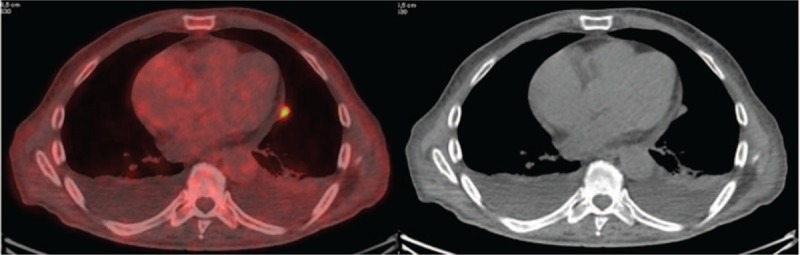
^18^F-FDG-PET/CT scan: small uptake in the left paracardiac area at the anterior segment of the lower lobe of the left lung not shown by ^68^Ga-DOTATOC PET/CT scan.
